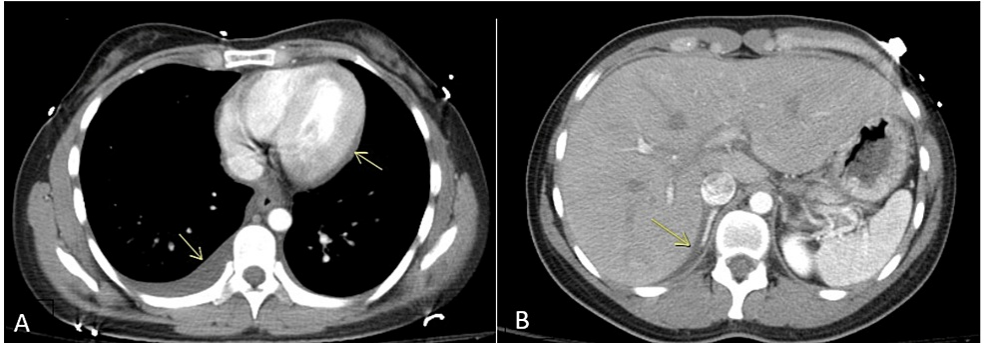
A computerized tomography of the abdomen and pelvis demonstrating pleural effusions and intra-abdominal fluid. (A) Sagittal views of a CT demonstrating a small right basilar pleural effusion, as denoted by the yellow arrow, as well as a pericardial effusion, which is also denoted by another yellow arrow. (B) Sagittal views of a CT demonstrating a small amount of perihepatic fluid, as denoted by the yellow arrow.
